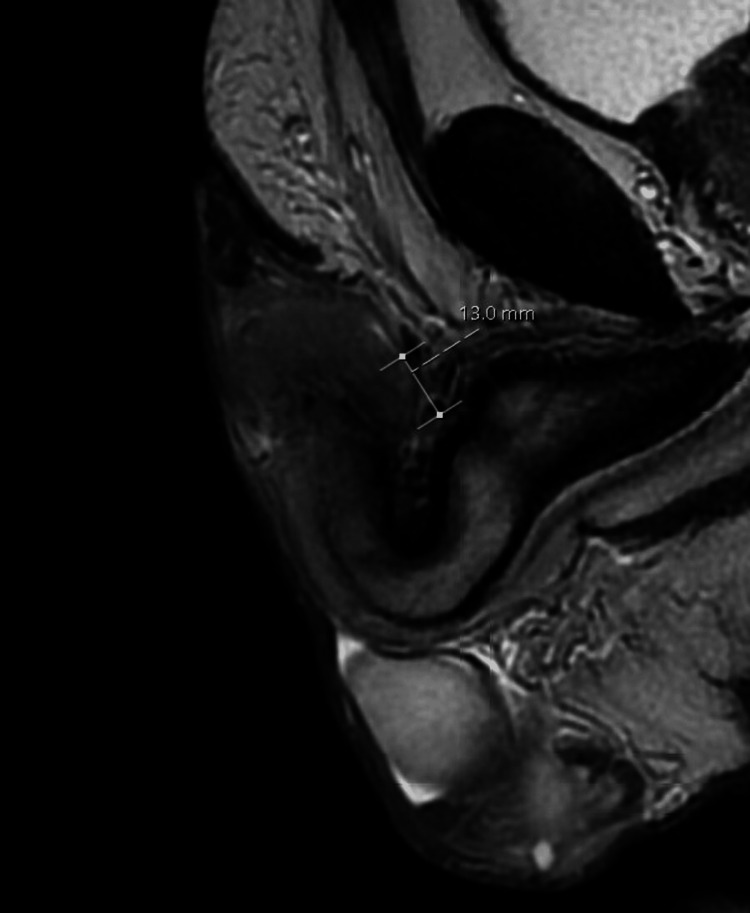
Sagittal MRI plane (T2-weighted image) showing the lesion, measuring 13 mm
